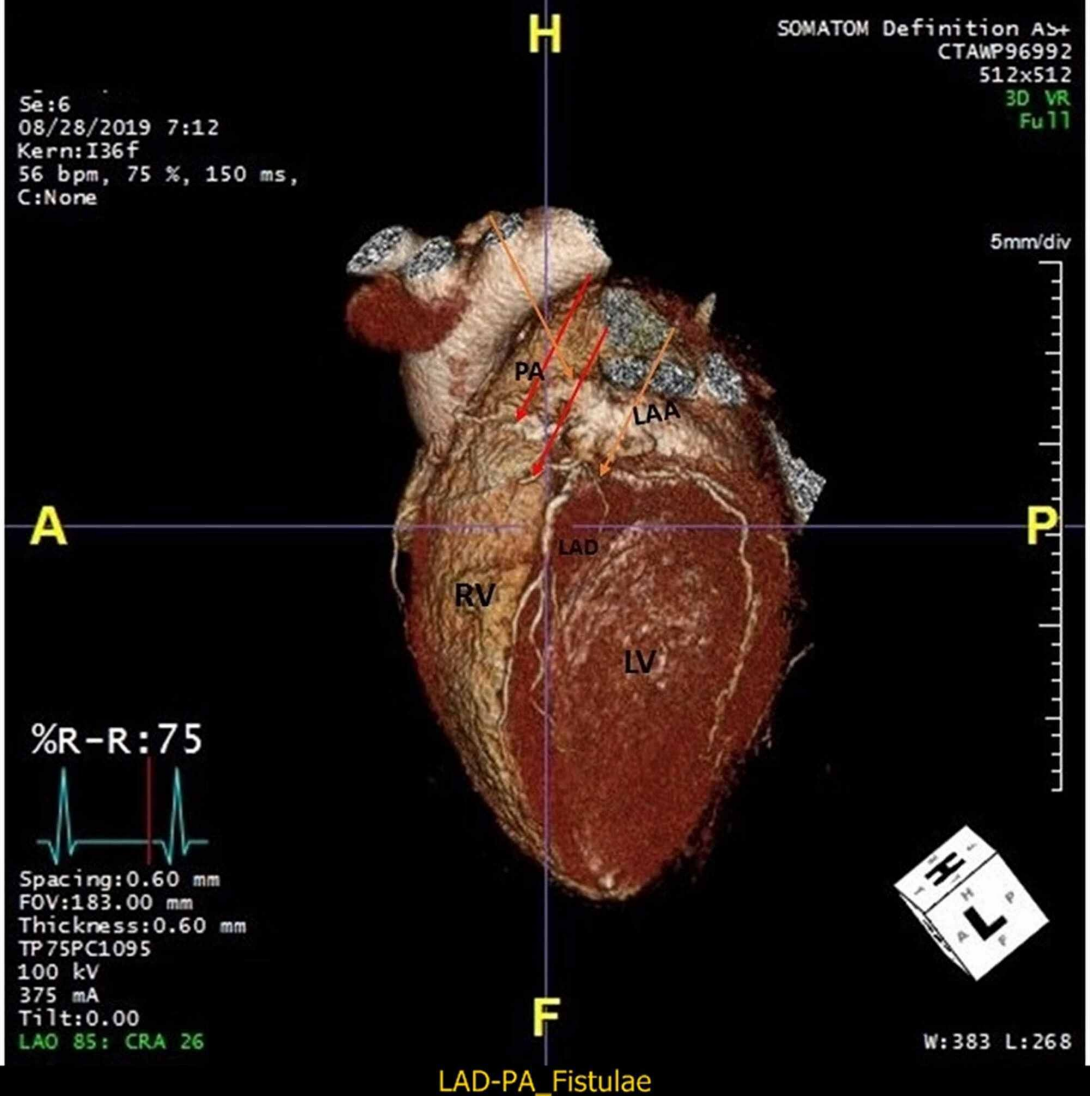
Cardiac CTA image showing CAF 3D image showing multiple proximal LAD fistulae draining into left atrial appendage and pulmonary artery. (Red and orange arrow). CAF= Coronary artery fistulae, LAD= left anterior descending
